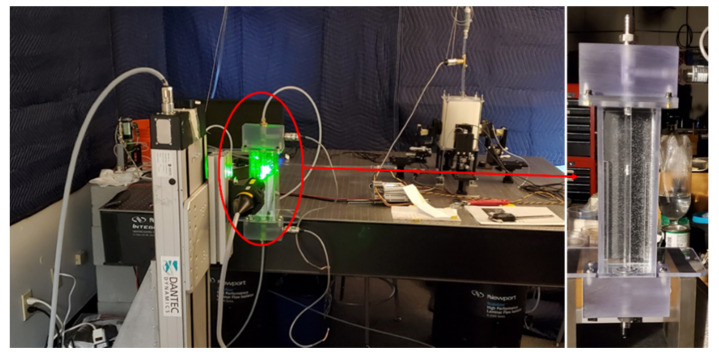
Experimental setup of Laser Doppler Anemometry (LDA) axial velocity measurements for a constricted pipe representing arterial stenosis.
